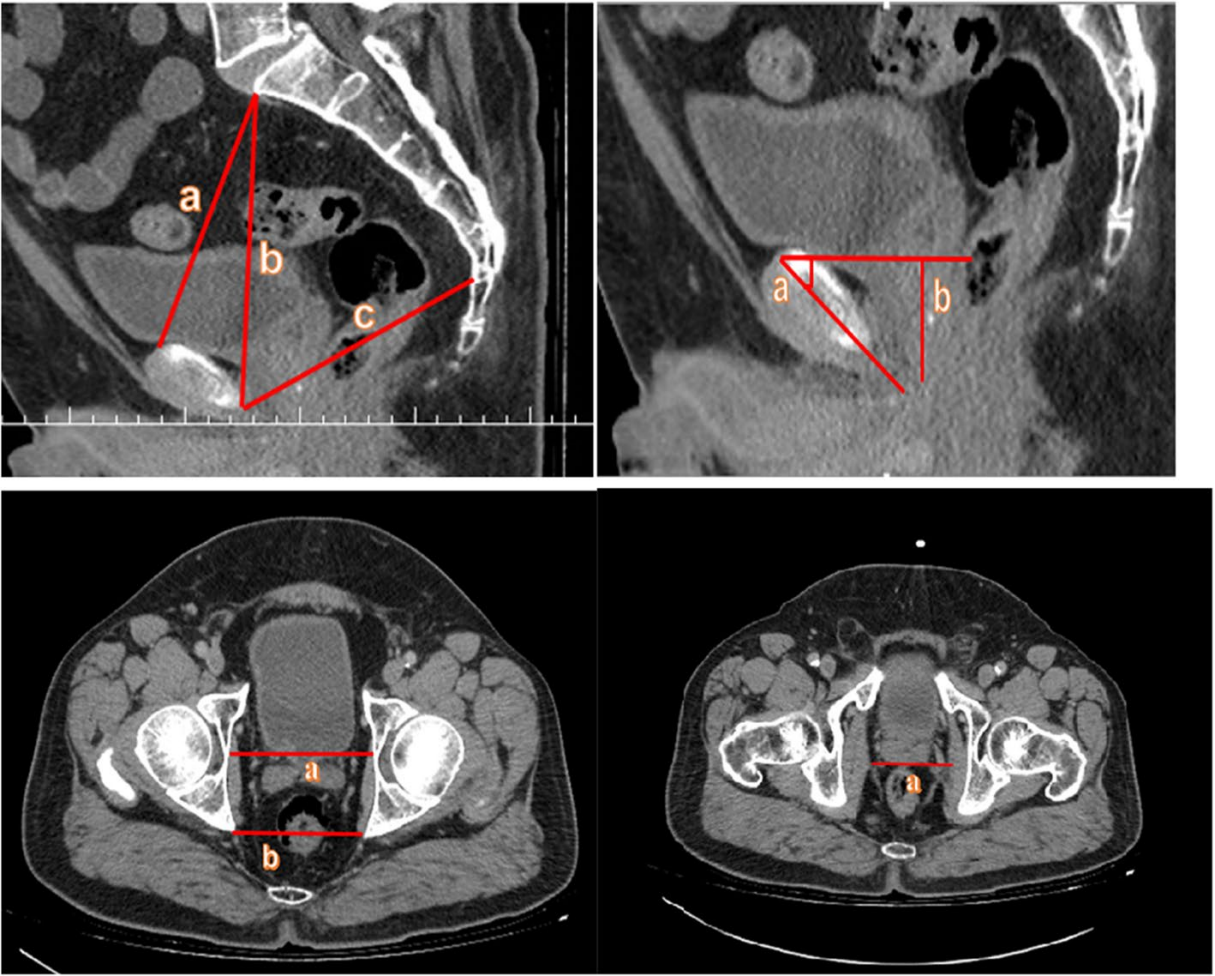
Upper left A) the upper conjugate (UC) was defined as the distance from the innermost aspect of the top of the symphysis pubis to the sacral promontory on the mid-sagittal plane; B) the pelvic depth (PD) was described as the distance between the promontorium and the lower symphysis pubis; C) The lower conjugate (LC) was defined as the distance from the lower symphysis pubis to the sacrococcygeal junction determined on the mid-sagittal plane. Upper right A) The symphysis angle (SA) was described as the angle between the long axis of the symphysis and the horizontal midsagittal plane. B) The apical depth (AD) was described as the distance between the highest point of the symphysis to the prostatic apex down left. Down left A) Bone femoral width (BFW) was mesasured as the bony width of the pelvis at the mid femoral head level. B) Interspinous distance (ISD) was measured on the axial plane between the tips of the ischial spines. Downright A) The soft tissue width (SW) was measured as the narrowest distance between the levator muscles on the axial CT images
